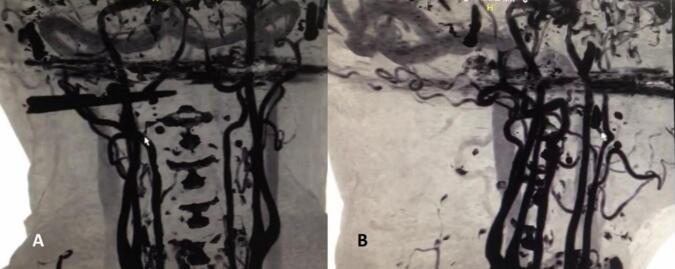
CT Angiography which showed the jaw was struck in the middle of patient's neck vessels between internal and external carotid artery.
